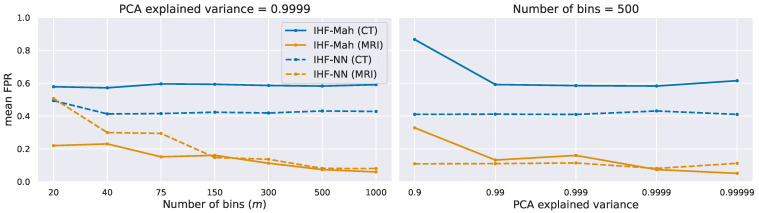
Dependence of IHF on its two hyperparameters: the number of histogram bins (*m*) and explained variance in PCA (*v*). We give the results for both IHF variants and CT and MRI setups.
